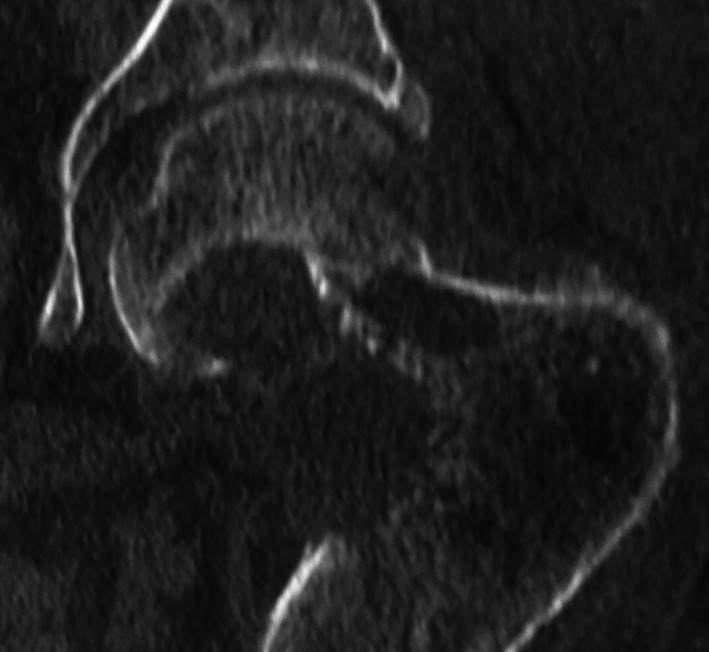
CT scan of the head and femoral neck: lytic bone lesions with cortical rupture and soft tissue invasion.
